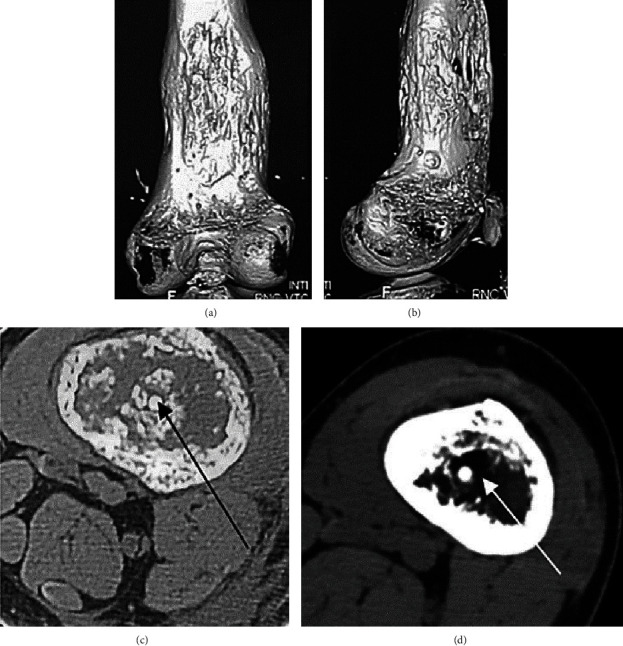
CT after frame removal: (a, b) 3D reconstruction of the bone regenerate in the distal femur upon frame removal; (c) transverse section of the distraction regenerate showing (arrow) exact position of the implant into newly formed bone at frame removal; (d) at 6-month follow-up.
